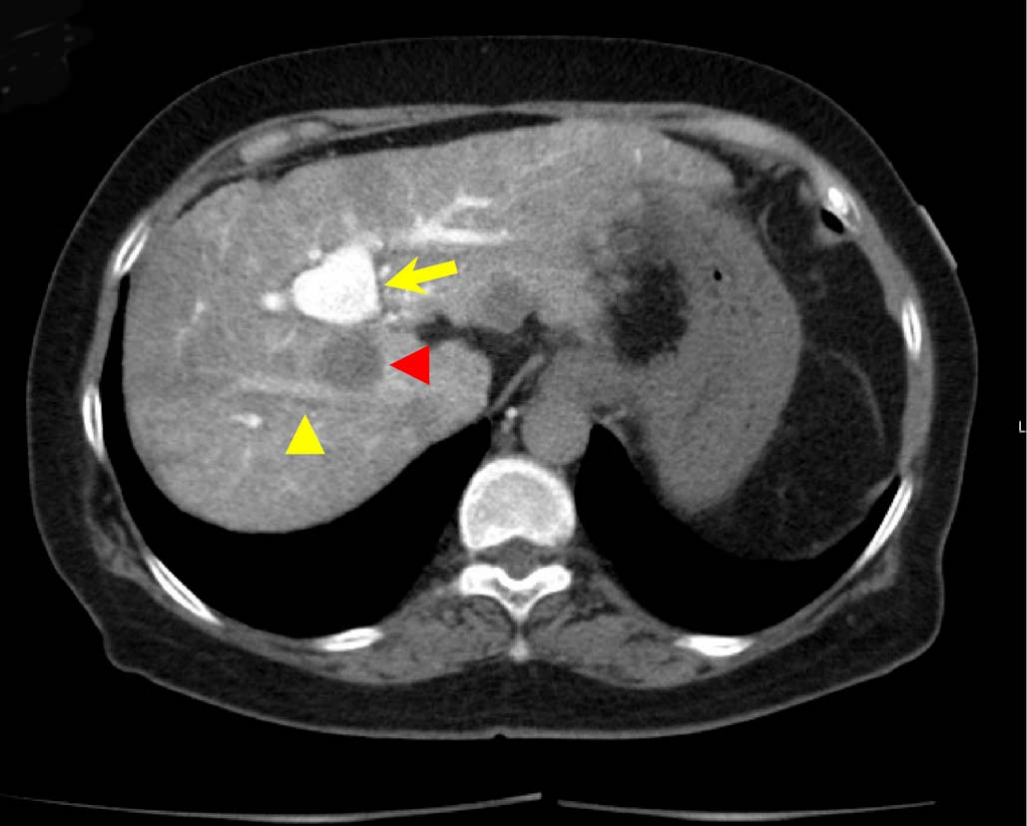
Computed tomography scan showing the hepatocellular carcinoma nodule (red arrowhead) adjacent to the intrahepatic B8 bile duct (yellow arrowhead). The high-density lesion observed above the hepatocellular carcinoma nodule (yellow arrow) is the transcatheter arterial chemoembolization scar.
